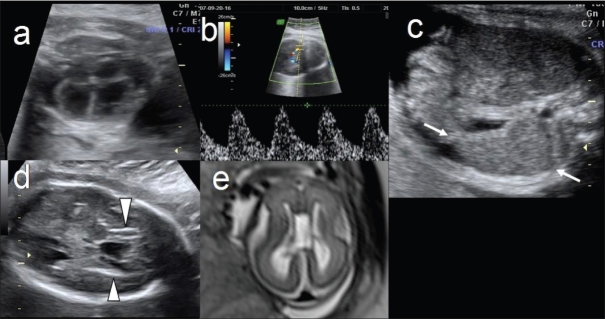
Pseudo-TORCH syndrome. Fetal cardiomegaly (a), elevated middle cerebral artery velocity (greater than 1.5 MoM) (b), fetal splenomegaly (arrows in c) and fetal periventricular calcification (arrowheads in d) are seen. Fetal cranial MRI (e) shows cerebral atrophy. All these features coupled with a negative maternal serology for infection, are diagnosticFetal toxoplasmosis syndrome: (transplacental infection by a protozoan, *Toxoplasma gondii*) Typical features of this syndrome would include hydrocephalus and cerebral calcification. Other features include microcephaly, hepatosplenomegaly, and ascites [Figure 10]. Confirmation is by PCR detection of the infective agent in amniotic fluid.
